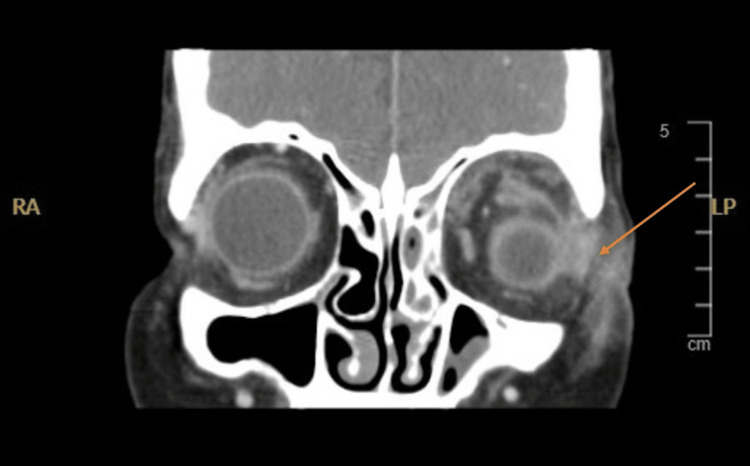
Enhanced coronal CT scan of the brain and orbit reveals opacified left pan-sinuses and mild pacification of right paranasal sinuses (including maxillary, frontal, and ethmoid air cells) and an enhanced collection in the left orbital region (arrow) along with the lacrimal gland
